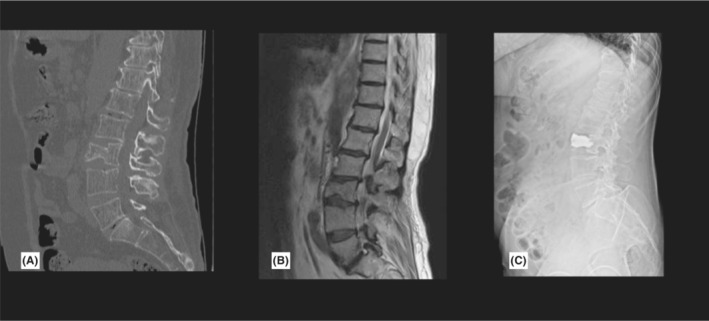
L3 burst fracture with negligible canal compromise in MDCT (A) and MRI (B). Post kyphoplasty x‐ray showed proper vertebral expansion and augmentation(C).
